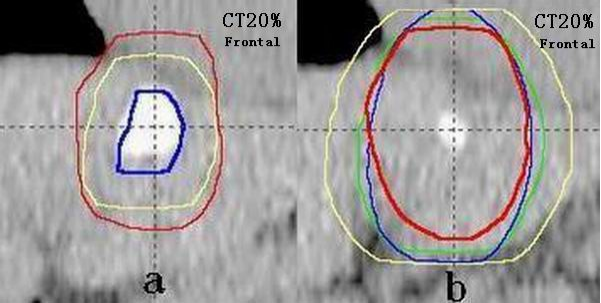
Example (Patient 1) of the different target volumes: (a) the ${\text{GTV}}_{3D}$ (blue line) and ${\text{CTV}}_{3D}$ (yellow line) derived from 3D CT, and ${\text{ITV}}_{4D}$ (red line) derived from 4D CT; (b) four PTVs based on 3D CT and 4D CT – ${\text{PTV}}_{4D}$ (red line), ${\text{PTV}}_{\text{conv}}$ (green line), ${\text{PTV}}_{\text{spec}}$ (blue line), and ${\text{PTV}}_{\text{vector}}$ (yellow line).
